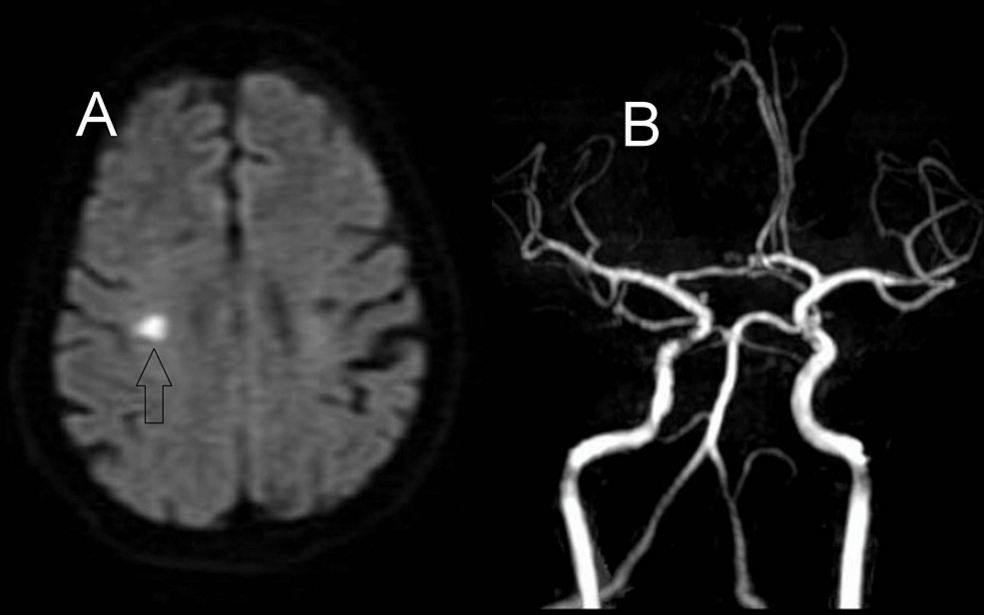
MRI and MRA study images (A) Magnetic resonance imaging (MRI) of the brain diffusion-weighted image (DWI) sequence showing acute infarct (arrow) in the right precentral gyrus. (B) The magnetic resonance angiography (MRA) of the brain was normal.
